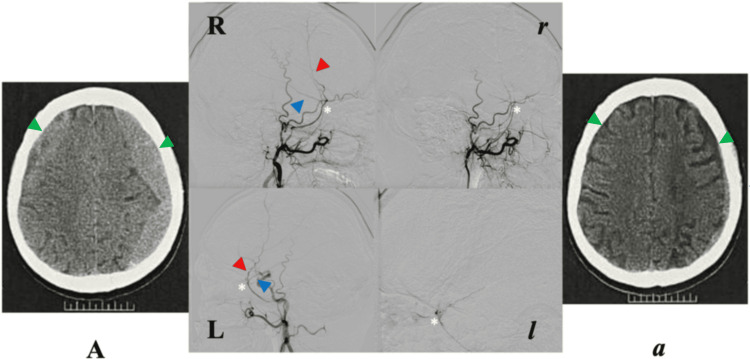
Case A. Pre-EMMA (A) and respective post-EMMA (a) cranial NCCT axial views at levels of maximum CSDH thickness. Pre-EMMA angiographies in lateral projections (R, right; L, left) show the course of anterior and posterior MMA branches, and post-EMMA obliteration of these vessels (r, right; l, left). Angiographic projections correspond to contiguous NCCTs. EMMA, embolization of the middle meningeal artery; L, left; MMA, middle meningeal artery; NCCT, non-contrast computed tomography; R, right. Green arrowhead, chronic subdural hematoma; red arrowhead, anterior branch; blue arrowhead, posterior branch; white asterisk, MMA bifurcation point.
